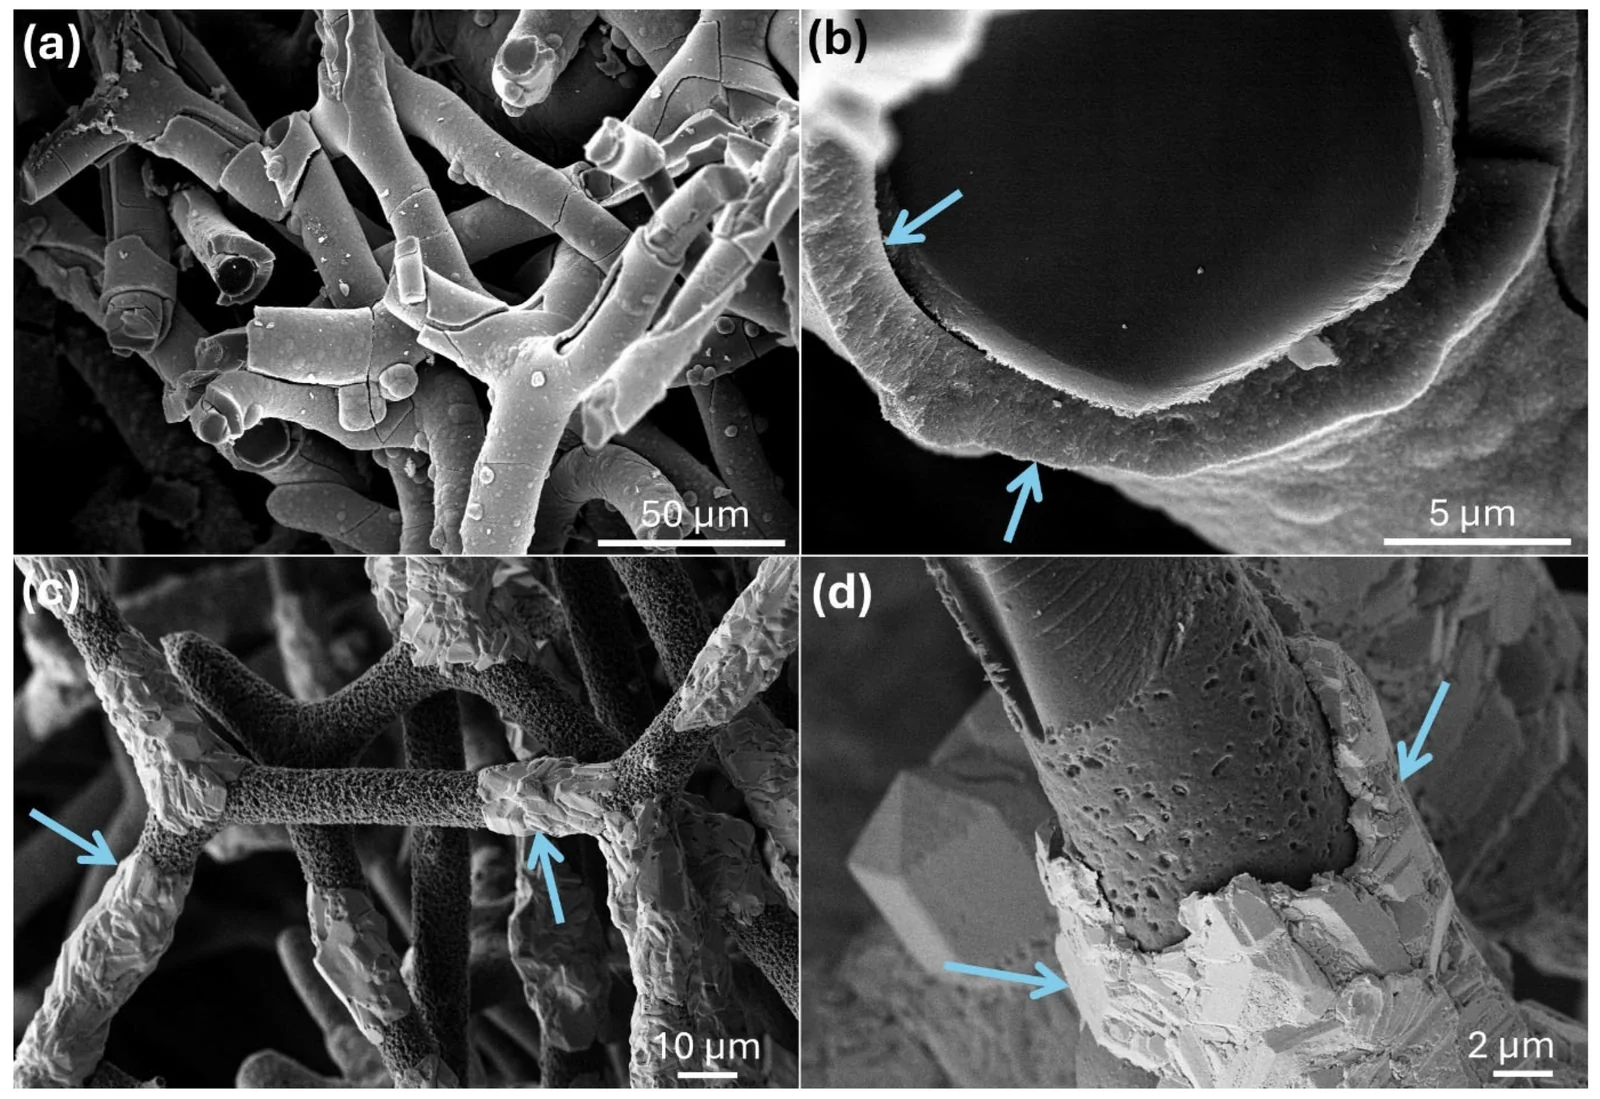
SEM imagery: carbonized spongin fibers of *H. communis* demosponge origin hydrothermally covered with a microlayer identified as MnO_2_ (a) remaining stable ((b) arrow) even after 1 h of ultrasonic treatment (for details, see [13]). Turbostratic graphite-based 3D scaffolds from the same sponge species can also be covered with up to 1 µm thick layers of copper oxides ((c,d) arrows) using electroplating.
